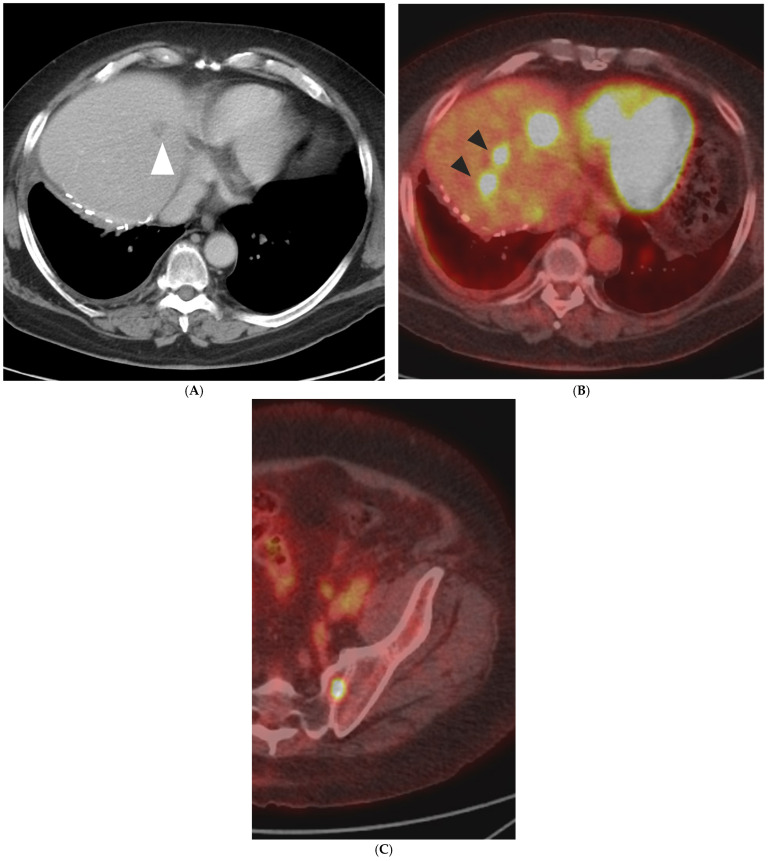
A 75-year-old man with metastatic rectal cancer, prior hepatic arterial infusion pump chemotherapy, and right hepatectomy, referred to interventional radiology clinic for consideration of percutaneous ablation to new 1.7 cm hepatic dome metastasis on CT ((A); white arrowhead). Prior to evaluation in clinic, an FDG PET/CT was obtained showing additional sites of intrahepatic disease ((B); black arrowheads), as well as osseous metastases ((C); left sacrum). The plan for ablation was aborted and systemic chemotherapy was administered.
